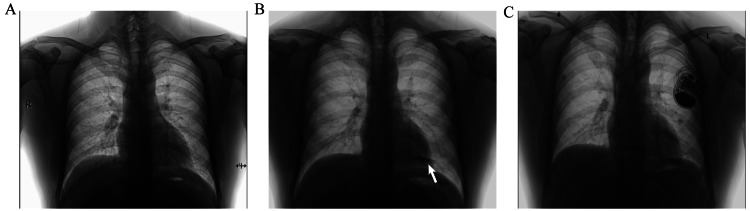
Chest X-ray A: normal chest X-ray; B: after implantation of an implantable cardiac monitor; C: after implantation of an implantable cardioverter defibrillator.
